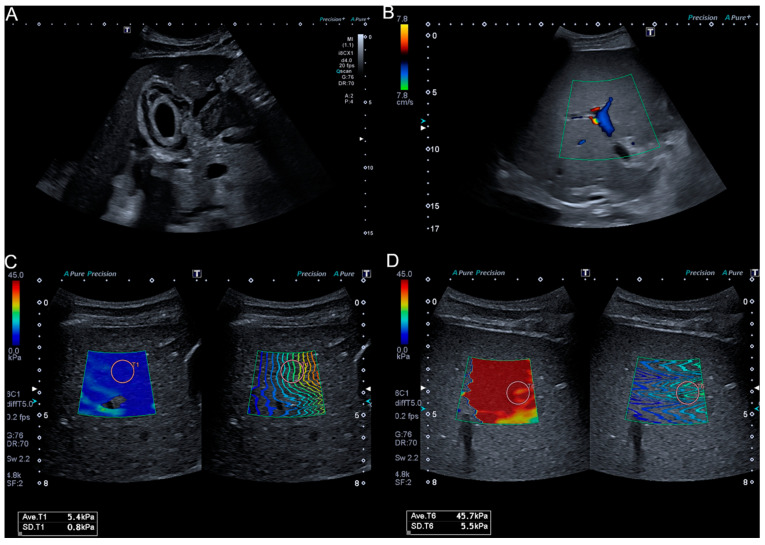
US imaging and SWE in a 36-year-old SOS/VOD patient. Gallbladder wall-thickening (A); reversed portal vein flow in color Doppler ultrasound (B); 2D-SWE before HSCT (C); and after development of SOS/VOD after HSCT (D).
